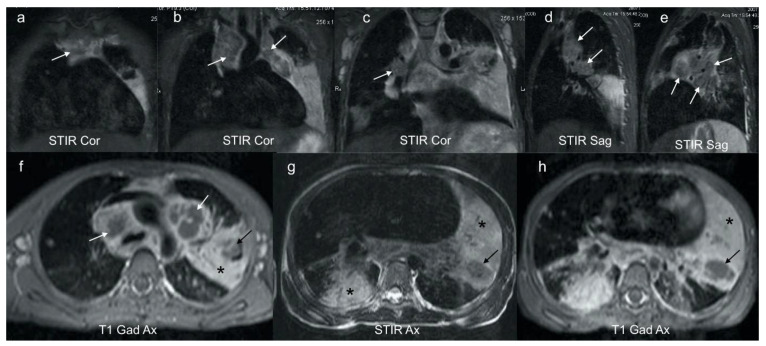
(a–h): Coronal, sagittal and axial MRI in a 6-year-old girl with confirmed pulmonary TB. Coronal (a–c) and sagittal STIR (d,e) images demonstrate characteristic low signal TB lymphadenopathy (white arrows) in the right and left paratracheal, and hilar regions. These can be compared with the higher signal axillary lymph nodes in image (c) which represent the appearance of non-TB nodes. Axial post gadolinium T1 at the level of the aortic arch (f) demonstrates that the STIR low signal lymphadenopathy in (a–e) demonstrates rim enhancement (white arrows), typical of centrally necrotic TB nodes. In addition, there is homogenous enhancement of a dense left consolidation (star) with an area of non-enhancing low signal (black arrow), in keeping with parenchymal breakdown within the consolidation. The axial STIR (g) and corresponding gadolinium enhanced T1 (h) at the lower zone of the lungs demonstrates an intermediate-to-high STIR signal enhancing consolidation posteriorly on the right (star) in keeping with viable lung; an intermediate STIR signal, poorly and heterogeneously enhancing consolidation on the left (star) in keeping with at risk lung; and a T2 low STIR signal non-enhancing focal area on the left (black arrow) typical of TB necrosis (this is the opposite to the STIR signal of an abscess, which would be bright).
